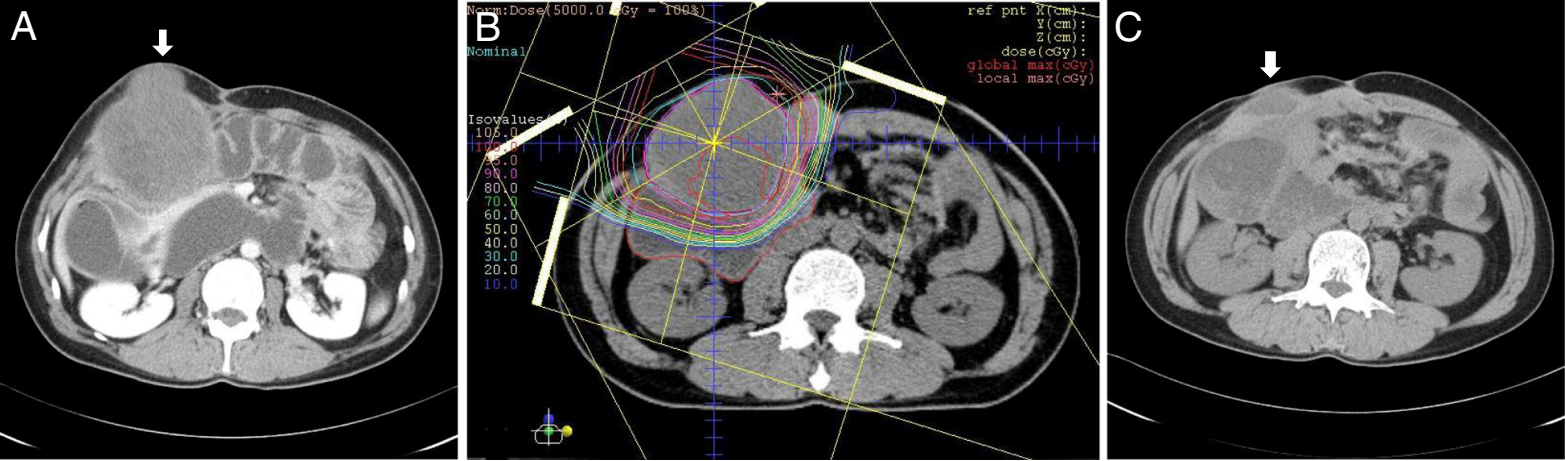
Abdominal computed tomography (CT) scan. CT scan revealed the desmoid tumor in abdominal wall (*solid white arrow* in a and c). a Pretreatment phase, b Carbon ion treatment plan and c 3 years after ion radiotherapy were shown
